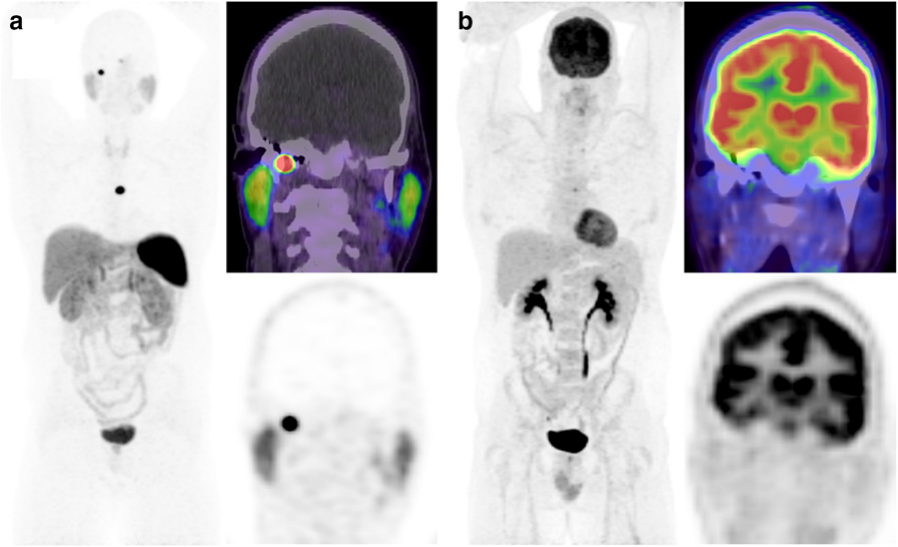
A 43 year-old male with *SDHD* germline mutation and prior excision of multiple paragangliomas. PET imaging was performed to characterise an equivocal mediastinal nodal abnormality identified on CT. Ga-68 DOTATATE (a) demonstrate very intense uptake in a right skull base glomus jugulare tumour (SUVmax = 90), which was not visible on F-18 FDG, in part, attributable to high uptake in physiologic cerebral activity (b). A left paratracheal abnormality in the mediastinum showed intense DOTATATE uptake and low grade uptake on FDG. This likely represented a further paraganglioma rather than a metastasis. The presence of 2 tumour suggests a probable germ-line mutation and would have warranted genetic testing if the germ-line mutation was not already known
